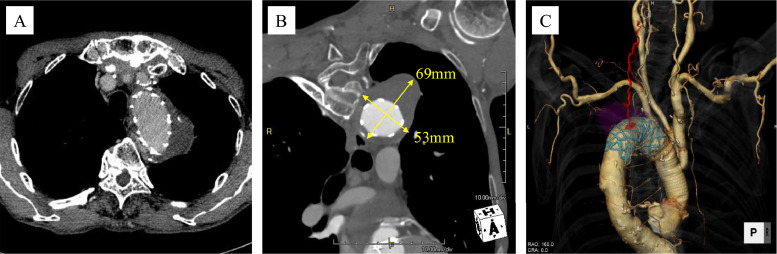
Computed tomography (CT) in Case 1. (A) A type II Endoleak (T2EL) demonstrated in the proximal descending aorta. (B) Maximum diameter of the aneurysm enlarged to 69 mm. (C) Feeding vessels originated from the left intercostal artery, deep cervical artery, costocervical artery, and left subclavian artery.
